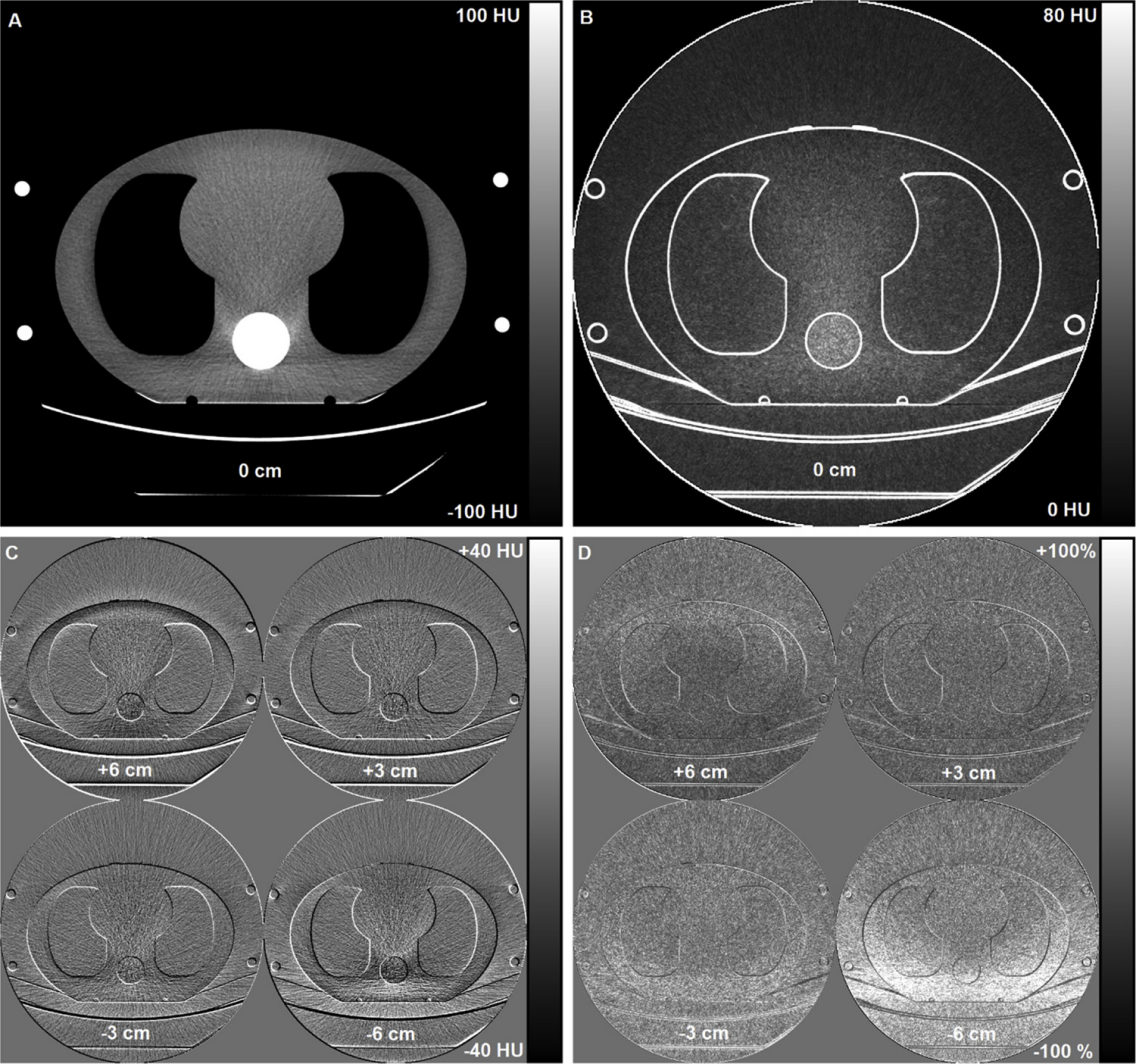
Axial image data from the GE chest CT scan with a fixed 120 kVp tube voltage after an AP scout. The reference axial CT image with mean CT numbers (A) and corresponding 1SD noise map (B) were calculated from ten slices comprising a total z-direction volume coverage of 10 cm. Absolute CT number differences (C) and relative noise difference maps (D) at table heights +6, +3, -3, and -6 cm were calculated relative to the reference centering (0 cm).
